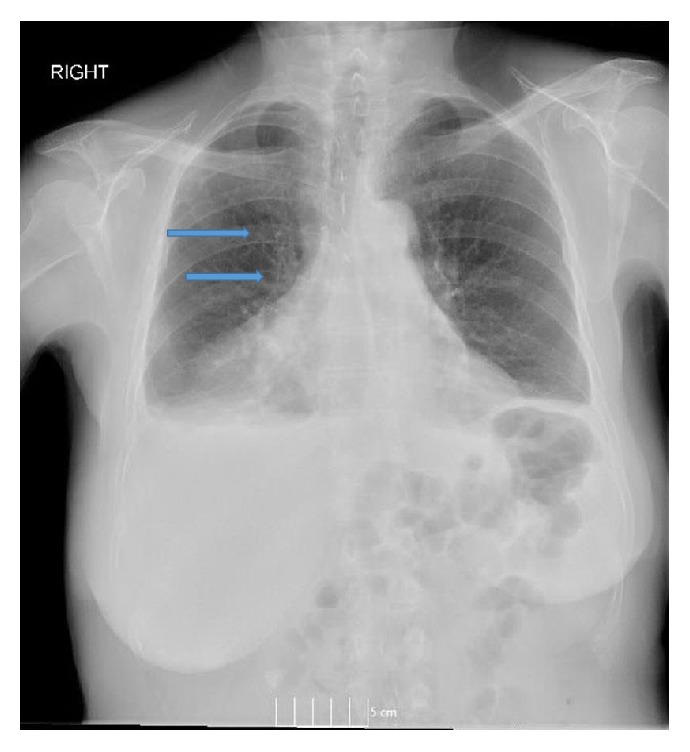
Chest X-ray (Posteroanterior view) showing bilateral pleural effusions with an expansile lucent lesions with septations in the right fourth and fifth ribs (arrow).
